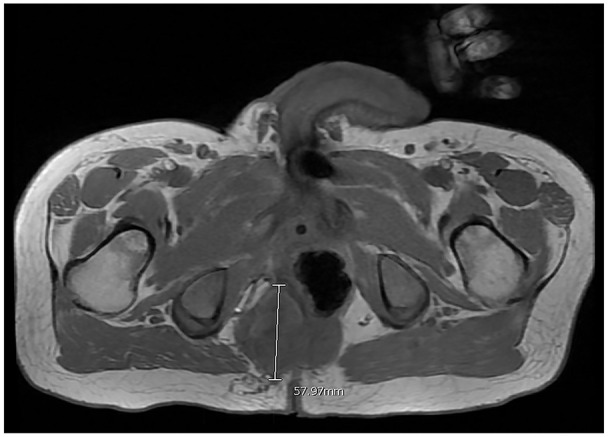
Magnetic resonance imaging (MRI) of the pelvis showing the axial view of the soft
